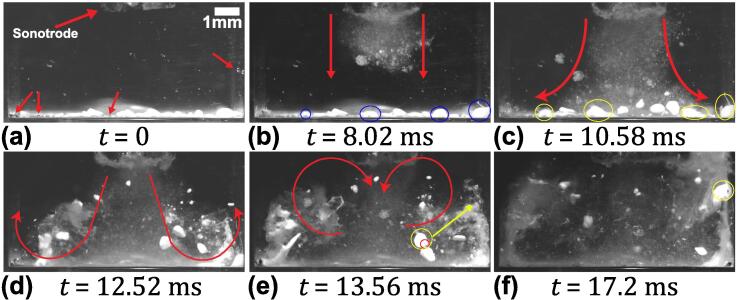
Sequence of images showing de-agglomeration and dispersion of fewer oxide aggregates under the influence of ultrasound captured at 50 kfps with a sonotrode amplitude of 210 μm peak-to-peak amplitude. See Supplementary video 2.
